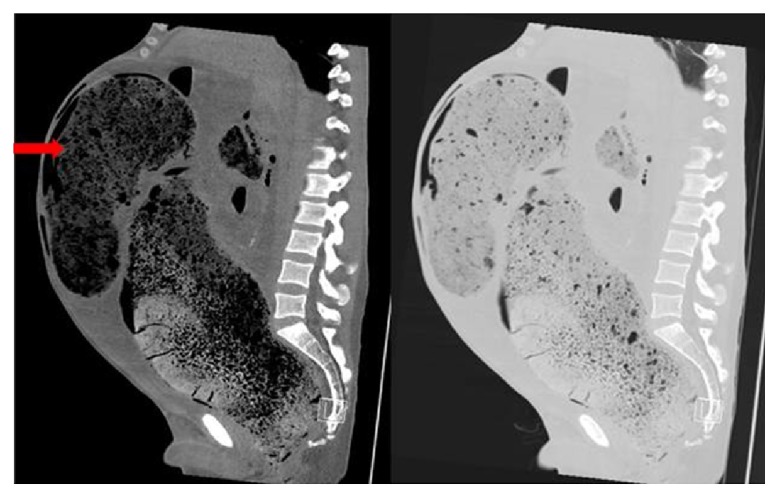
Sagittal MPR CT images with soft tissue windows on the left and lung windows on the right. There is gross chronic colonic distension with faecal loading down to the low rectum. The arrow shows faeces in a capacious sigmoid colon. No free gas on the film.
